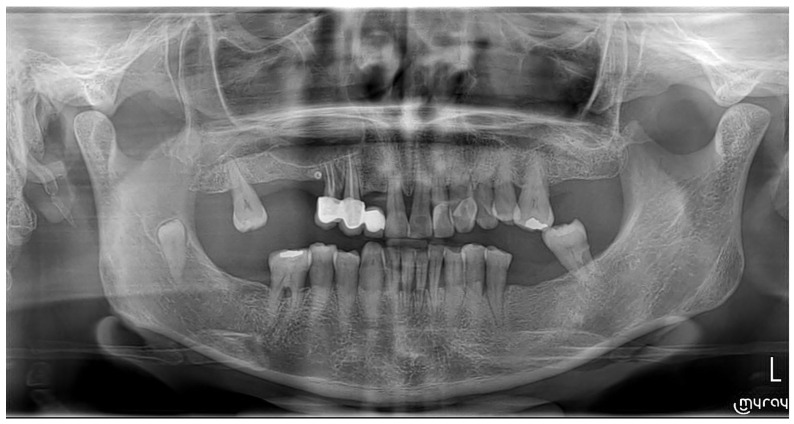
Two-year follow-up panoramic radiograph demonstrating stable bone healing in the region of the previously enucleated odontogenic cyst, with no evidence of recurrence.
